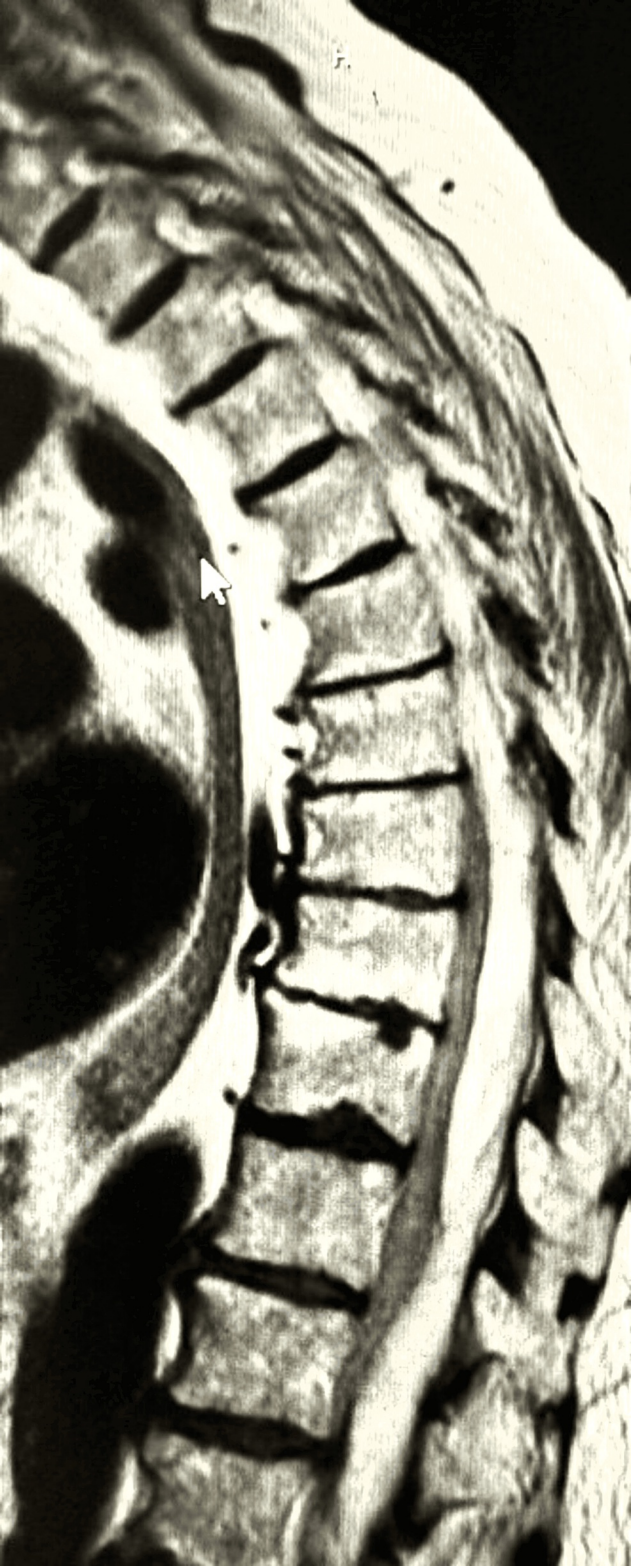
MRI revealing an epidural hematoma (arrow) within the posterior spinal canal extending from T6 to the lower sacrum, accompanied by fluid accumulation at T9 and T11 levels.
